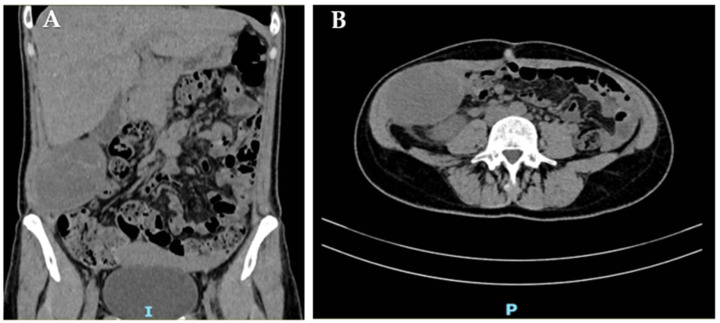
CT scan of the right abdominal wall: coronal (A) and axial (B) view of the abdominal-wall DT.
